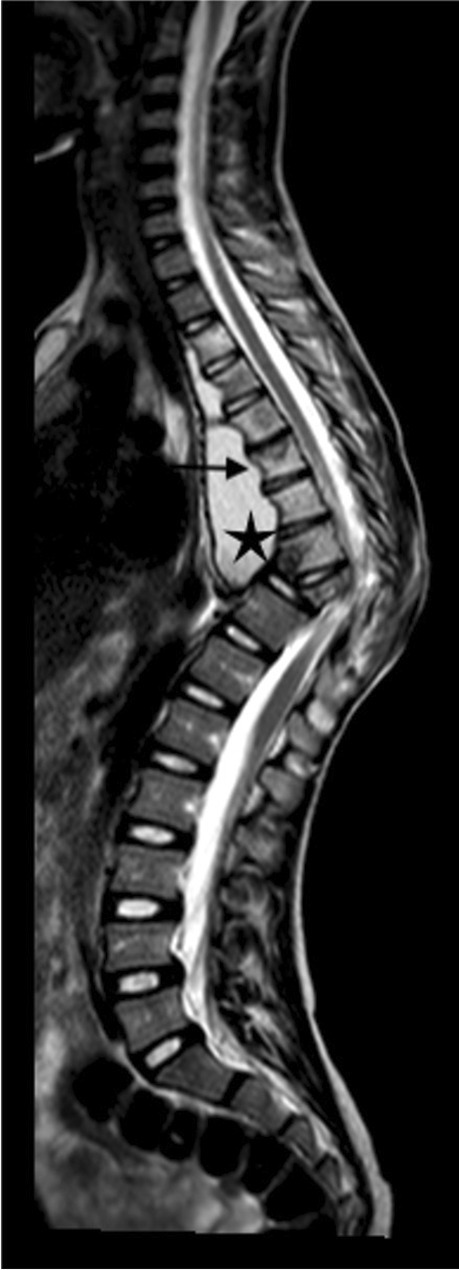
A 6-year-old boy with tuberculous spondylitis. Sagittal T2 magnetic resonance image of the spine shows scalloping of the anterior vertebral bodies (*arrow*) secondary to an anterior subligamentous collection (*asterisk*). There is a thoracic kyphosis due to collapse of the T10 vertebral body
